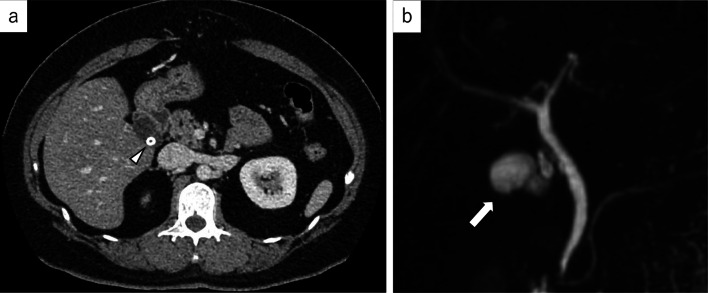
Contrast-enhanced CT of the abdomen and MRCP images. a Contrast-enhanced axial CT: a calculus is seen in the neck of the gallbladder (arrowhead). b MRCP: there are no common bile duct stones and no intrahepatic bile duct dilatation. The remnant gallbladder is indicated by the arrow
